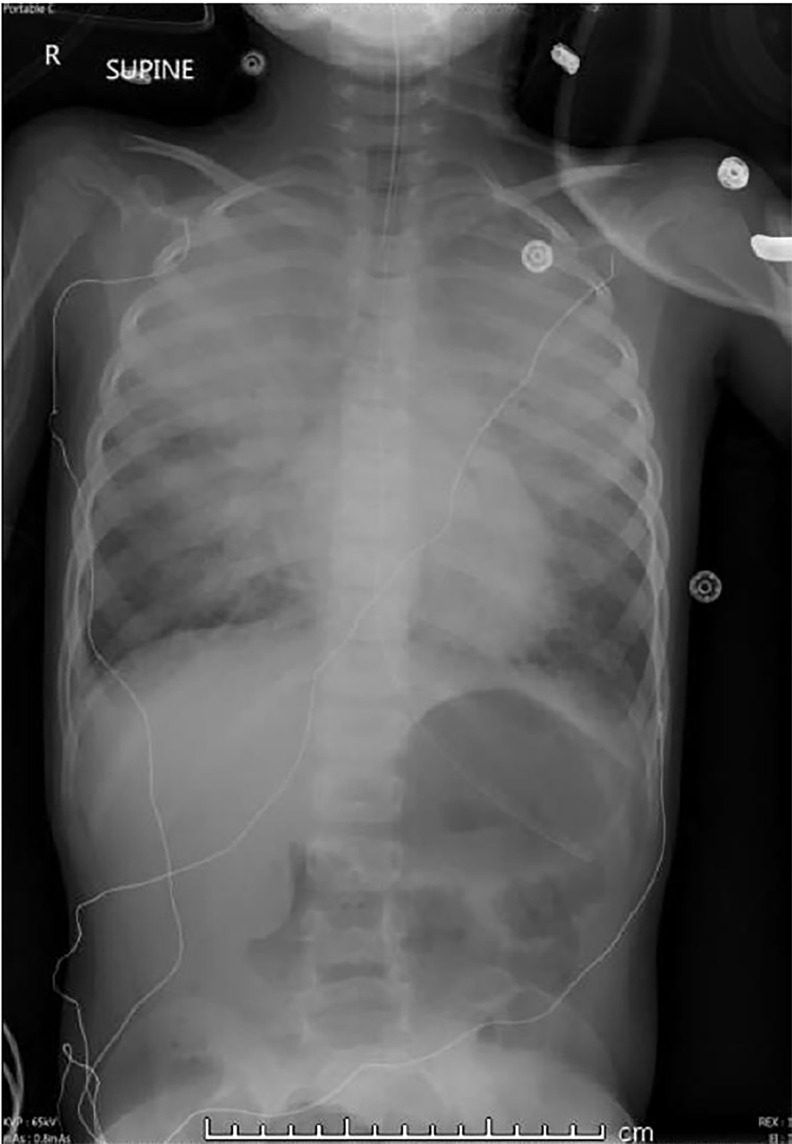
Post-Intubation chest radiograph demonstrating worsening edema consistent with acute respiratory distress syndrome.
